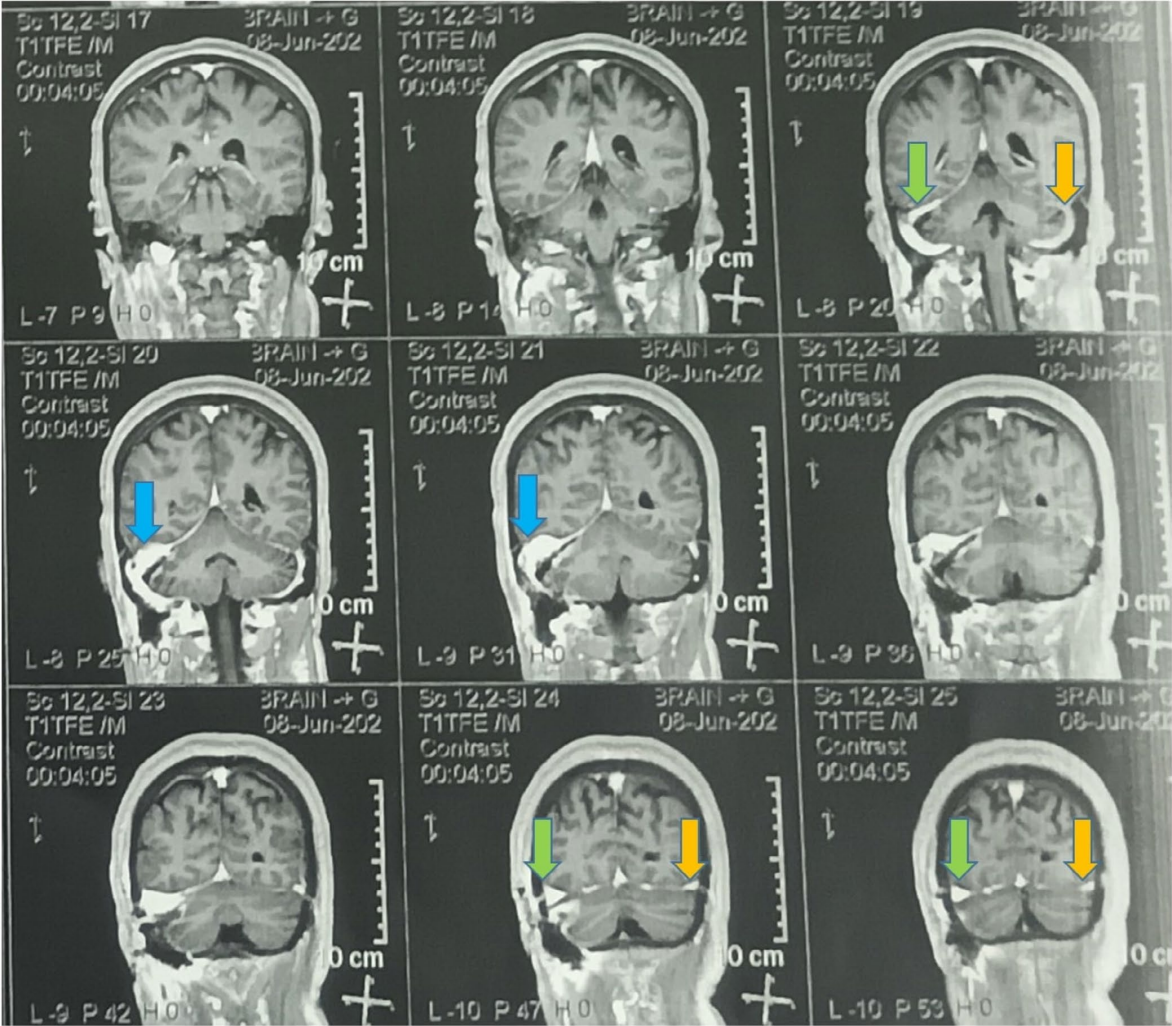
Postoperative brain MRI with Gad: The right transverse sinus (green arrow) is much more prominent than the left one (orange arrow), indicating the right dominant venous sinus system. The right sigmoid sinus (blue arrow) is dilated compared to the preoperative MRI.
